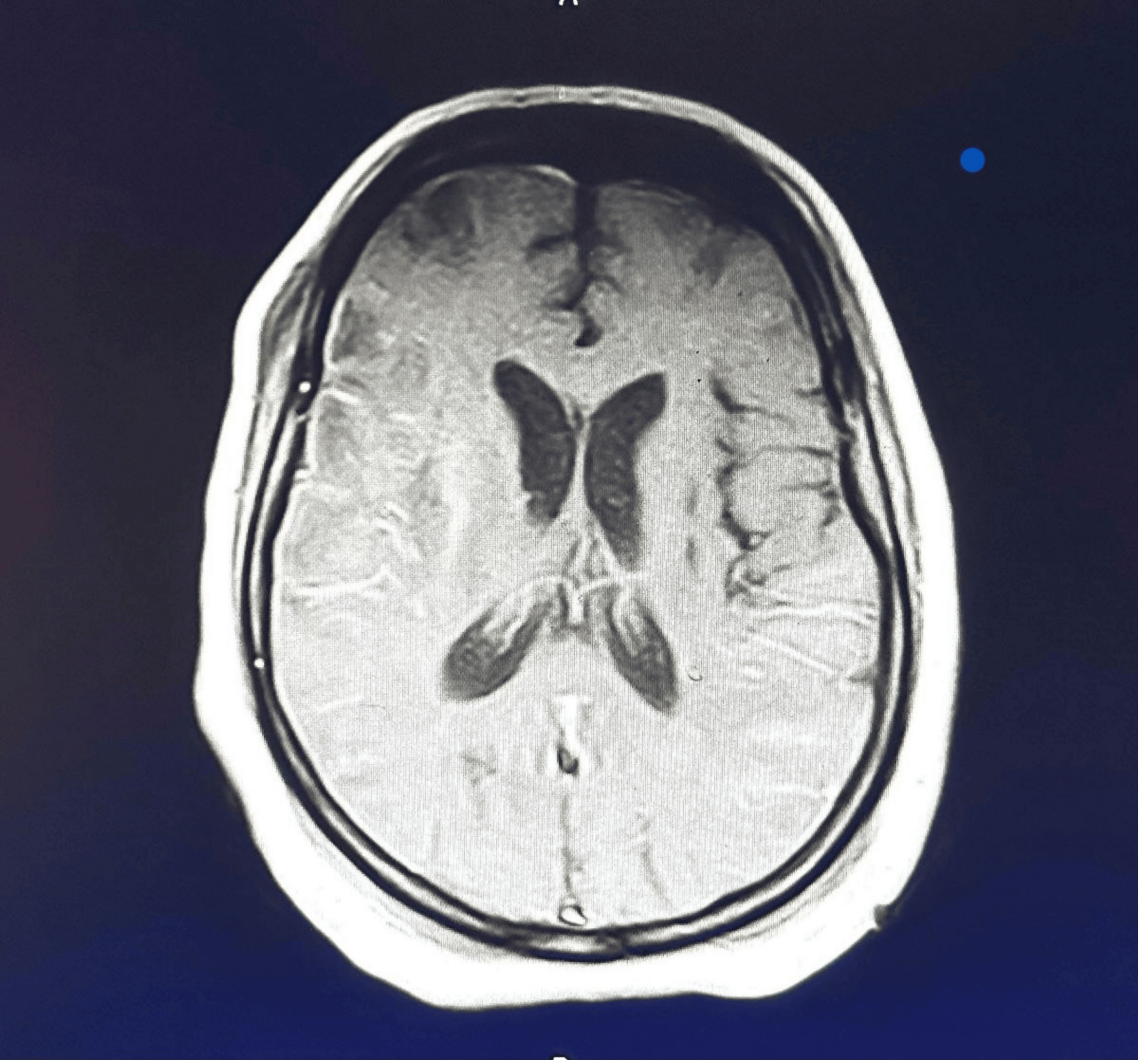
MRI of the brain shows ischemic changes.
